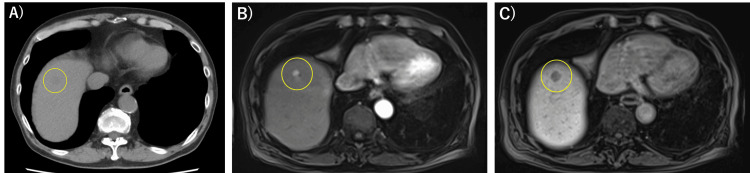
Plain CT and Gd-EOB-DTPA-Enhanced MRI Images (A) Plain CT image: a low-density mass was found in the right lobe of the liver (yellow circle). (B) Gd-EOB-DTPA-enhanced MRI (arterial phase) showing a 2-cm hyperenhancing lesion in segment 8 of the liver (yellow circle). (C) Hepatobiliary phase image demonstrating hypointensity of the same lesion (yellow circle). Gd-EOB-DTPA MRI: gadolinium-ethoxybenzyl-diethylenetriamine pentaacetic acid-enhanced magnetic resonance imaging.
